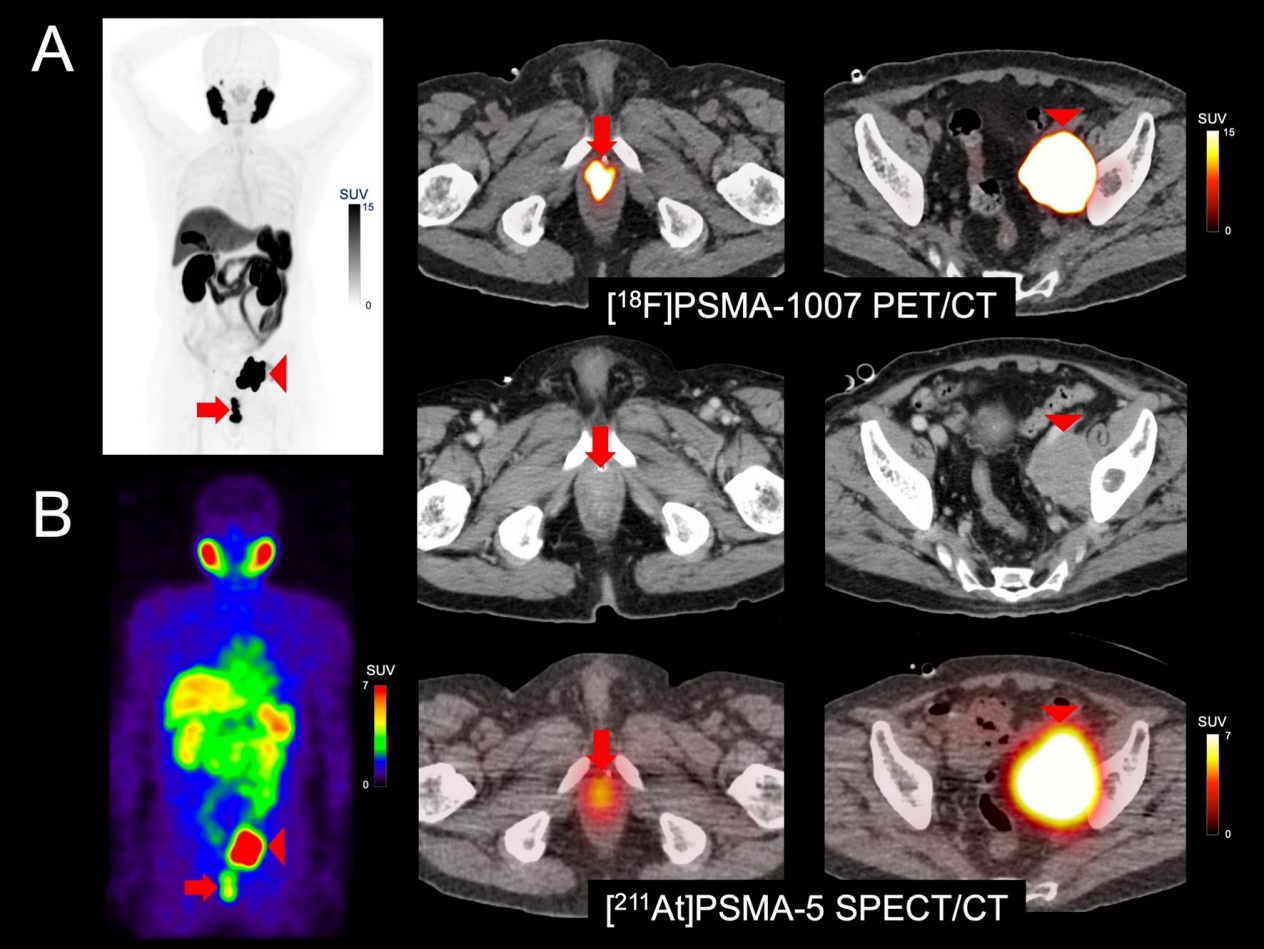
[^211^At]PSMA-5 was administered to a man in his 70 s with metastatic castration-resistant prostate cancer refractory to standard treatment, including androgen receptor signaling inhibitors, docetaxel, and cabazitaxel. Pre-treatment [^18^F]PSMA-1007 PET/CT (A) and [^211^At]PSMA-5 SPECT/CT (B) images showed similar distribution patterns, with high uptake in recurrent/metastatic lesions (left: maximum intensity projection, right: fusion and contrast-enhanced CT images). Both images revealed high accumulation in the soft tissue mass within the prostate area (SUVmax = 60.7 on [^18^F]PSMA-1007 PET and 4.9 on [^211^At]PSMA-5 SPECT) (arrows) and in the enlarged left external iliac lymph node metastasis (SUVmax = 143.7 and 17.6, respectively) (arrow heads). (Cited from reference no. 85 in accordance with the open access policy.)
